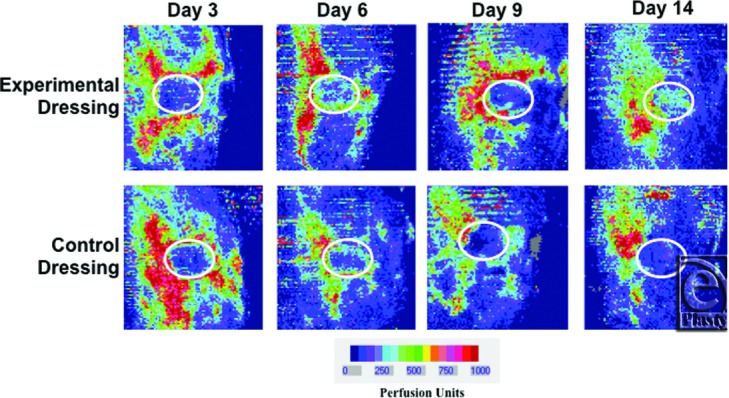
Laser Doppler imaging (LDI) used to quantify perfusion levels in experimentally treated versus control treated wounds. Representative LDI flux images from each time point are shown. White circles represent regions of interest where mean perfusion units were calculated for each wound.
